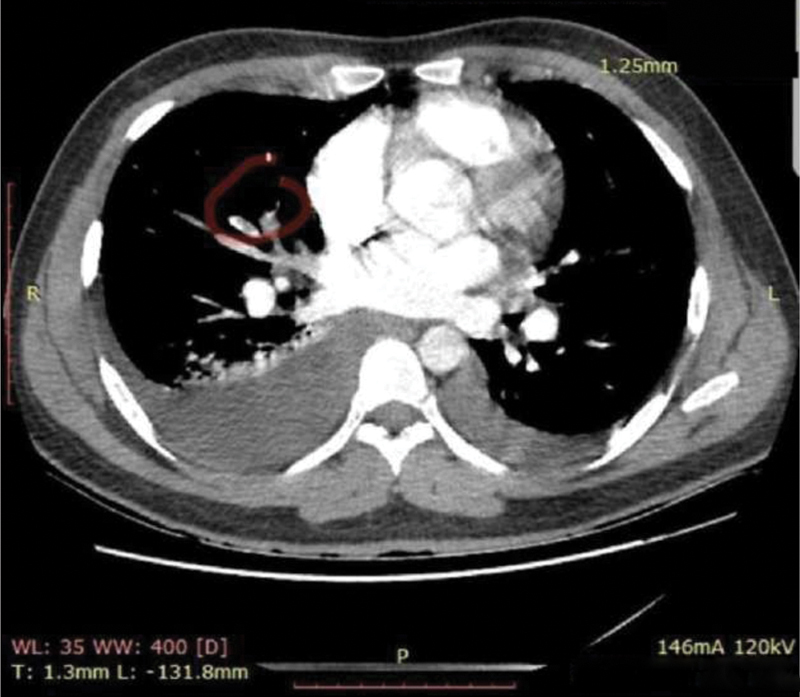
Chest computed tomography angiography demonstrating filling failure in the segmental artery of the right lower lobe (red circle).
